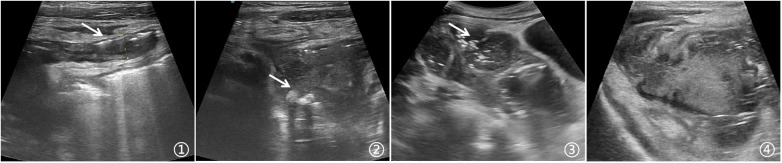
(1) The ultrasound can visualize the appendix with a roughly displayed appearance, and this interface shows the long-axis view of the appendix. The outer diameter of the appendix measures 0.97 cm. (2) A free appendicolith is visible within the abscess cavity. (3) A multi-loculated appendiceal abscess with multiple punctate gas echoes inside. (4) The abscess cavity is encapsulated, and the pus inside has a viscous consistency.
